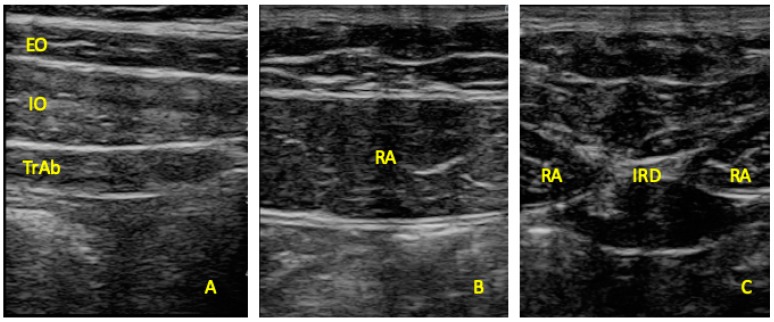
Ultrasound imaging thickness for the EO, IO, TrAb (A), RA thickness (B) muscles, and IRD (C). EO, external oblique; IO, internal oblique; TrAb, transversus abdominis; RA, rectus abdominis; IRD, inter-recti distance.
